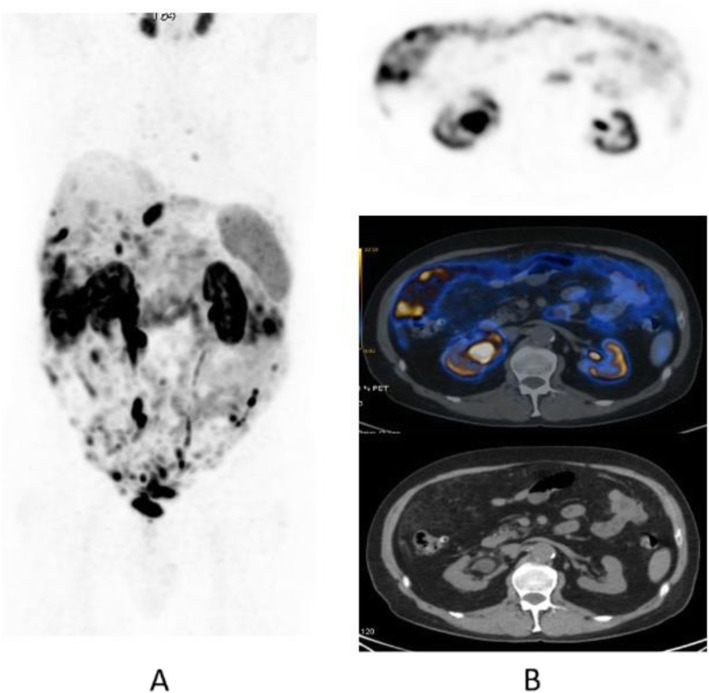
^68^Ga-PSMA, prostate cancer, biochemical recurrence (BCR): peritoneal invasion. *Clinical history*: 67 y.o. man with prostate cancer, radical prostatectomy as primary treatment Gleason score 4 + 3; during anti-androgen treatment, PSA increased up to PSA = 114 ng/ml. *PET/CT findings*: multiple foci of increased uptake in the peritoneum, liver capsule, and mediastinal lymph nodes. a MIP. b PET, fused, and CT images
